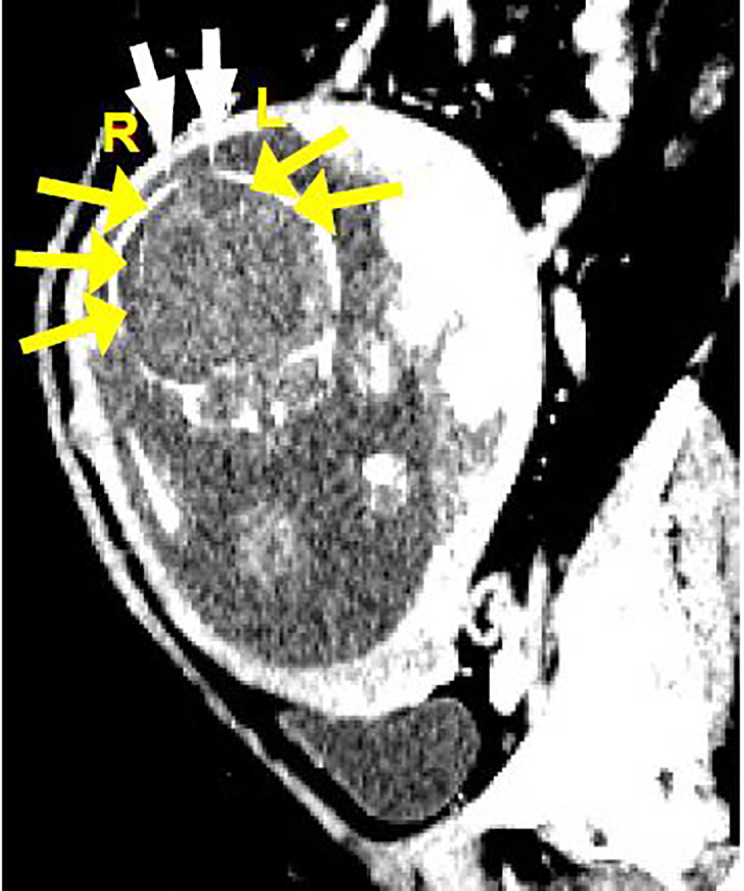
Computed tomography angiography abdomen and pelvis sagittal view revealed bilateral subdural hematomas (yellow arrows) and fetal skull diastasis (white arrows).
